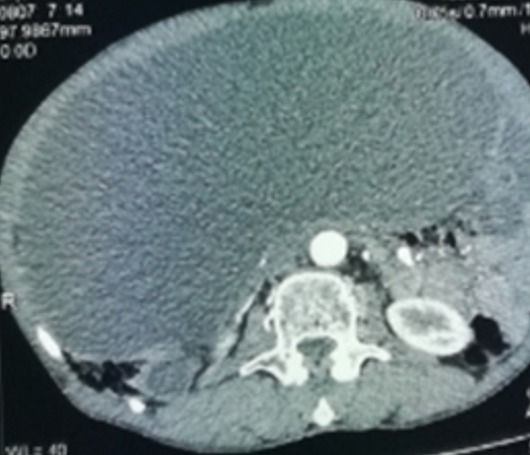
CT image of the hydatid cyst in its under mesocolic portion
